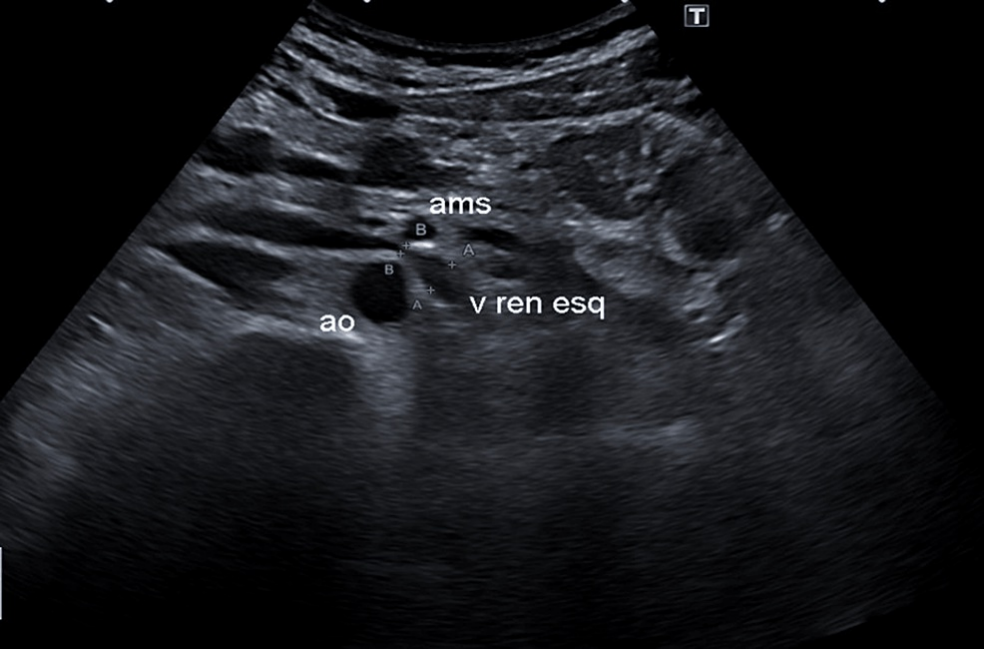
Doppler ultrasonography showing changes in the diameter of LRV between the AA and the SMA suggestive of NCS. LRV: left renal vein; AA: abdominal aorta; SMA: superior mesenteric artery; NCS: nutcracker syndrome
